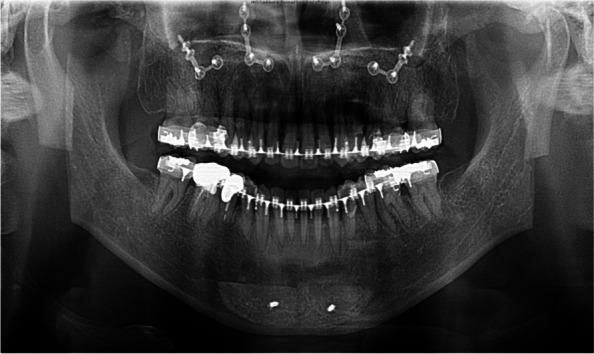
Panoramic radiography immediately after orthognathic surgery shows the inferior part of the proximal segment bones obtained after vertical ramus osteotomy being fixed onto the mentum of the mandible
